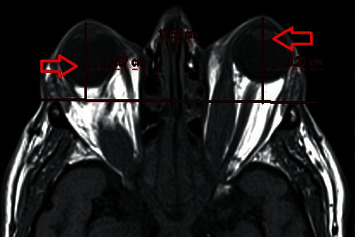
Exophthalmos measurement technique on MRI. The arrow shows the length from the foremost part of the cornea to the orbital apex.
